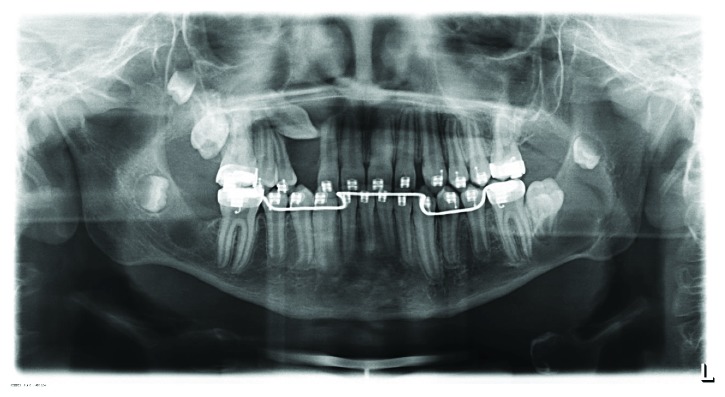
Follow-up panoramic radiograph (October 2015) shows a clear enlargement of the translucent area at tooth 13, as well as new changes associated to the crowns of teeth 18, 17, 37, 38, and 48.
